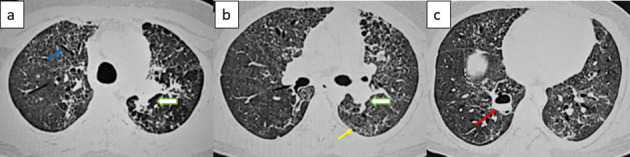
Axial HRCT chest image (a) reveal dilated bronchi (blue arrow); (b) inter- and intraseptal thickening predominantly in subpleural region(yellow arrow) and (c) small-sized thin-walled cavity with air-fluid level (red arrow). Focal patchy area of parenchymal opacification (white block arrows) was seen in the apicoposterior segment of left upper lobe.[Figure 2(b)]
